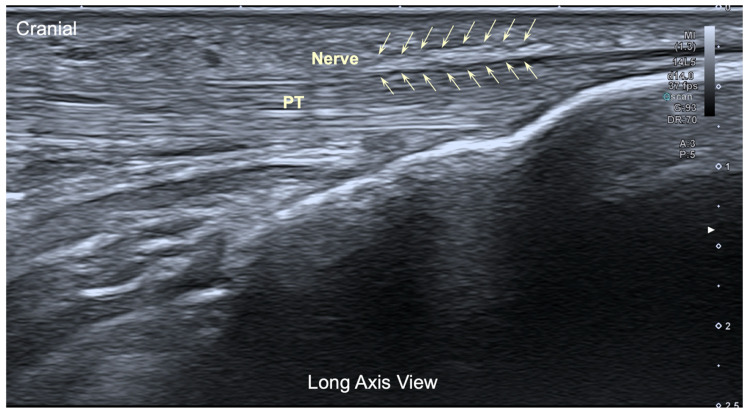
Ultrasound image (long axis view) of the infrapatellar nerve inferior branch. Pt: Patellar tendon.
